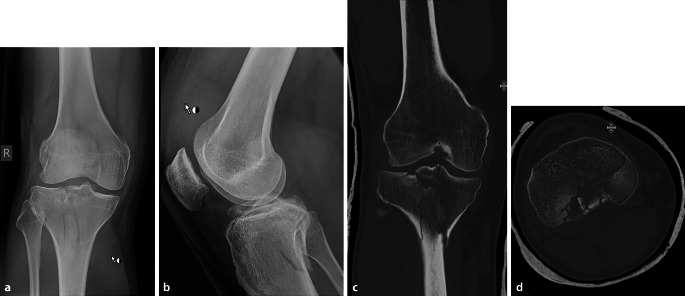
Aufnahmen eines 31-jährigen Patienten mit bikondylärer Tibiakopffraktur. Die initiale Versorgung erfolgte mittels Mecron-Schiene. Im Verlauf erfolgte dann die osteosynthetische Versorgung. a, b Röntgenbild anterior-posterior und lateral initial, c, d CT-Ausschnitt sagittal und axial nach Ruhigstellung in dorsaler Gipsschiene
